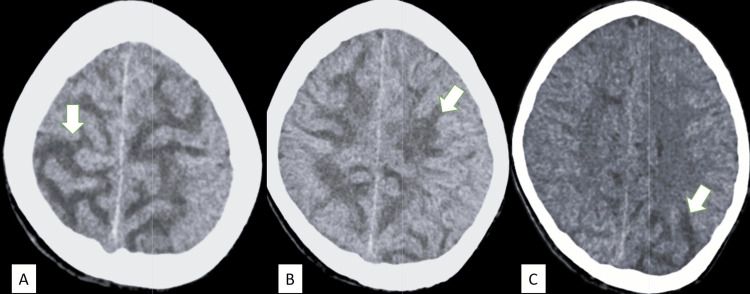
(A&B): CT head axial section at the centrum semiovale level showing bilateral symmetric hypodensities in white matter with persevered white-grey differentiation (arrows). (C): Section showing bilateral asymmetrical white matter hypodensities in the posterior parietal lobe (arrows), suggestive of vasogenic oedema.
